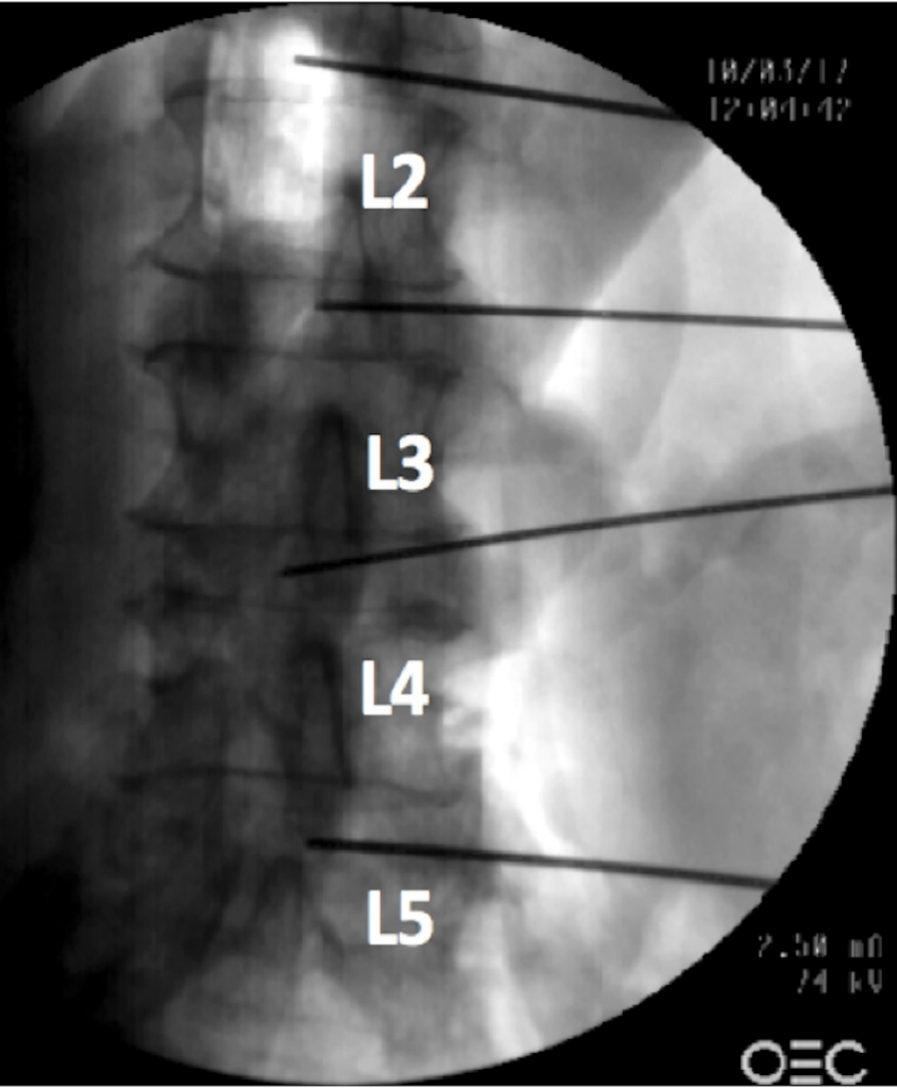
Labeled anteroposterior radiograph of the lumbar spine showing the placement of the wires which were used for the measurements.
